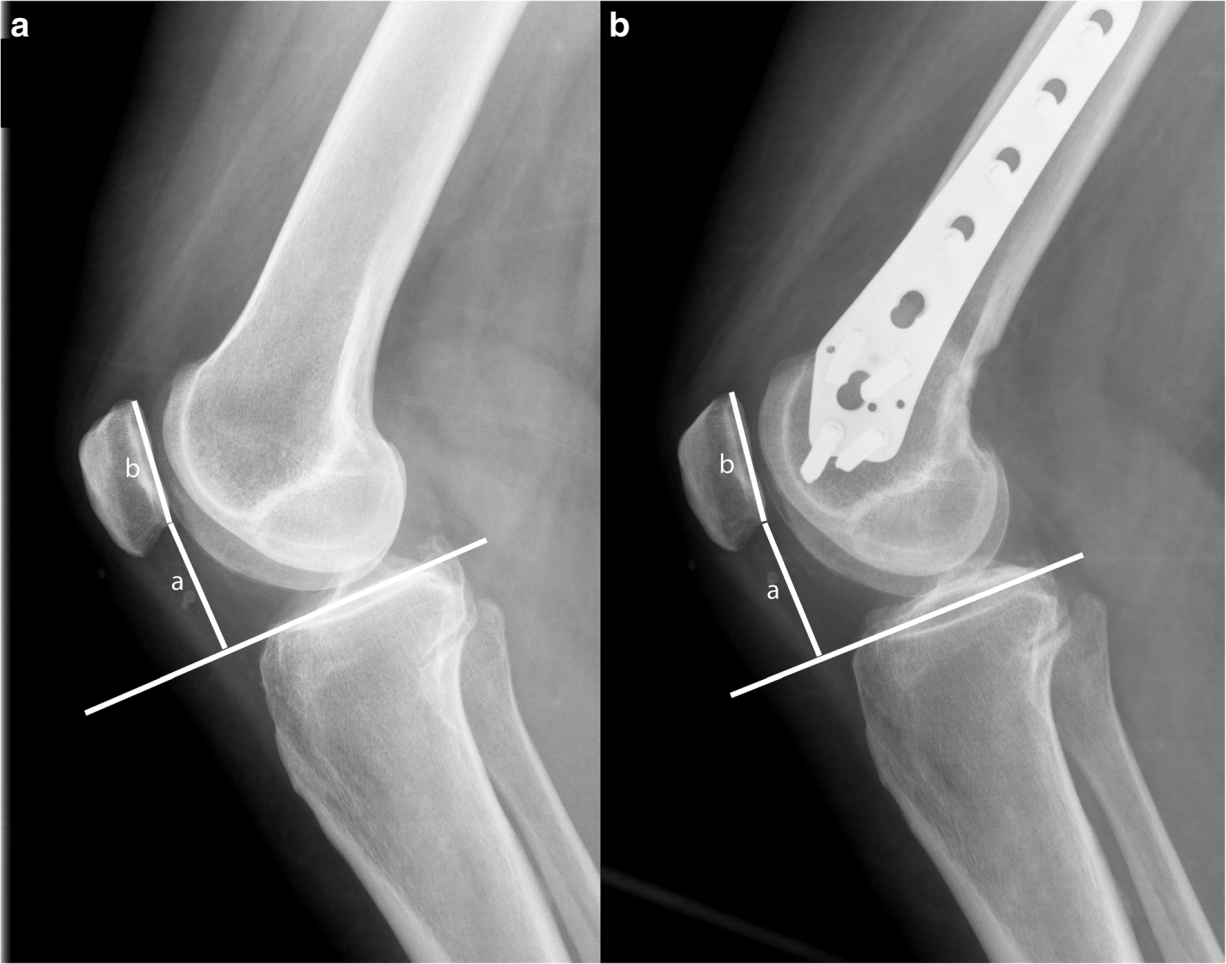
Measurement of the patellar height on lateral radiographs pre-operatively (a) and post-operatively (b); measurement of the perpendicular height of the lower end of the articular surface of the patella from the tibial plateau line (a) and length of the articular surface of the patella (b). The Blackburne-Peel ratio is defined as a/b [15]
